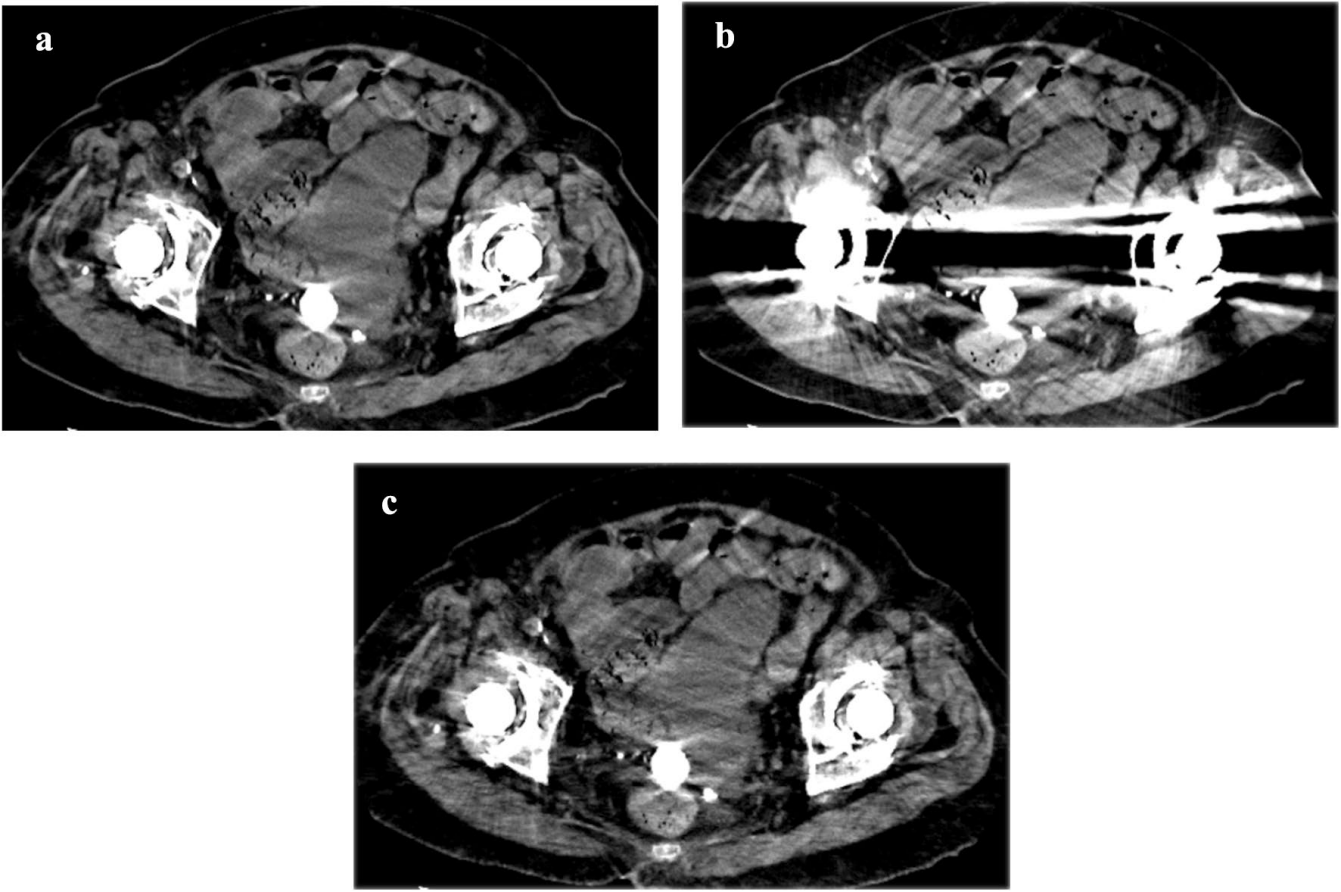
Axial unenhanced CT images reconstructed using DLR-S (a), DLR (b), and IR-S (c) of an 84-year-old woman. The degree of metal artifacts (DLR-S/DLR/IR-S) was rated as 4 (minimal artifacts)/1 (severe artifacts in a large area)/3 (moderate artifacts) by reader 1. It was rated 3 (moderate artifacts)/1 (severe artifacts in a large area)/3 (moderate artifacts) by reader 2. Bladder delineation (DLR-S/DLR/IR-S) was rated 5 (clear)/1 (unrecognizable)/2 (noticeably blurred) by reader 1. Reader 2 rated it as 4 (slightly blurred)/3 (moderately blurred)/4 (slightly blurred). A calcified structure was observed in all the reconstruction algorithms (a–c). However, the structure location was recognizable only on the DLR-S (a) and IR-S (c) images
